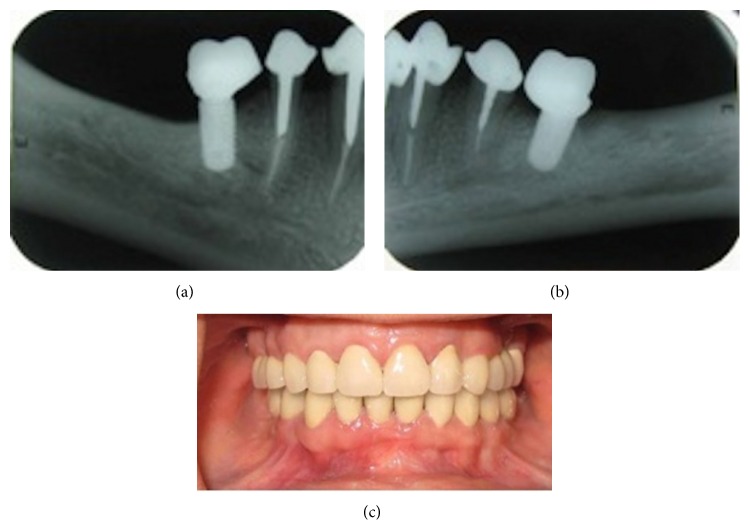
Intraoral view and periapical radiographies of implants after one-year follow-up.
